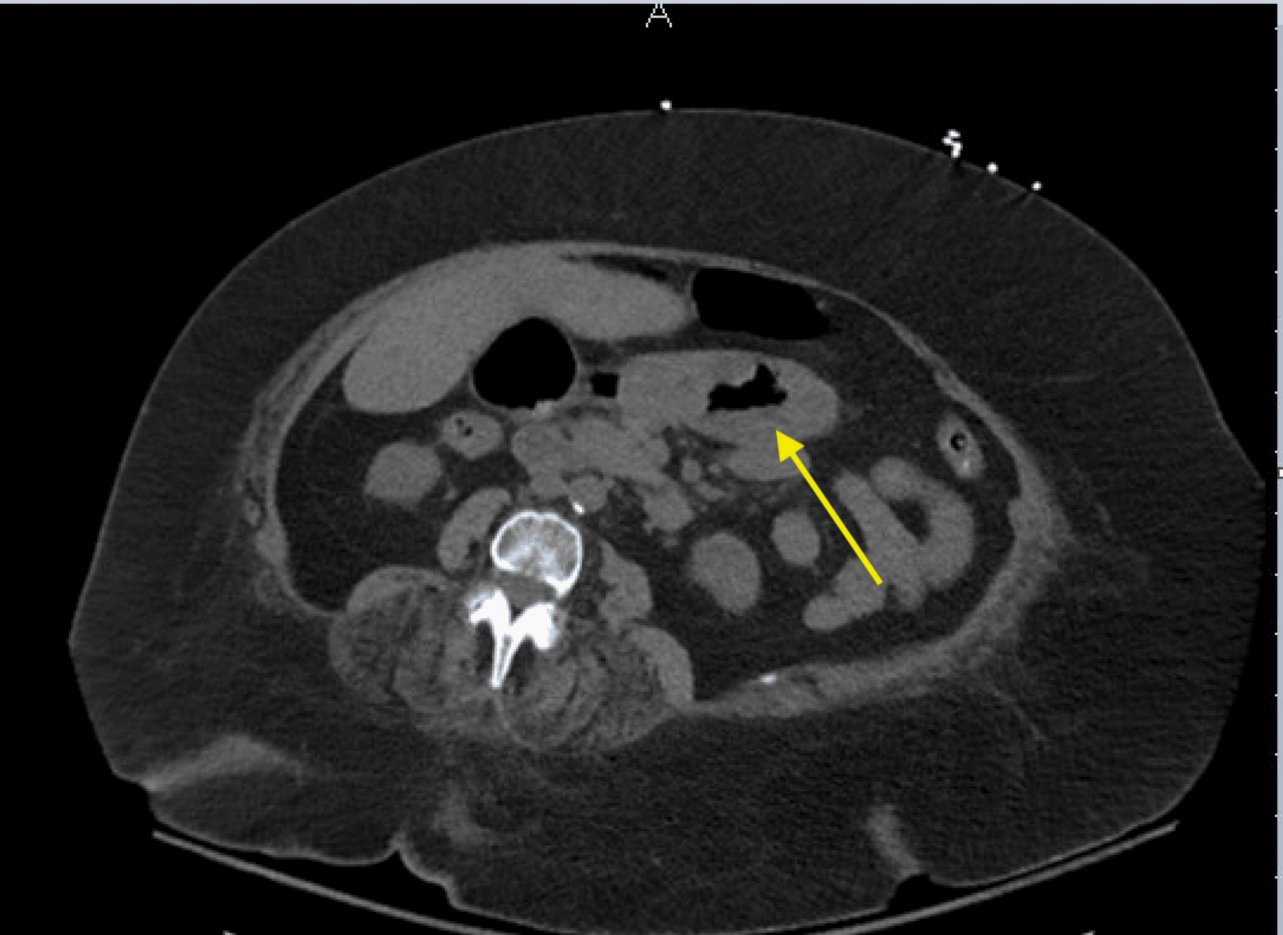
Computed tomography image of the abdomen/pelvis without contrast showing moderate gastric wall thickening (arrow)
